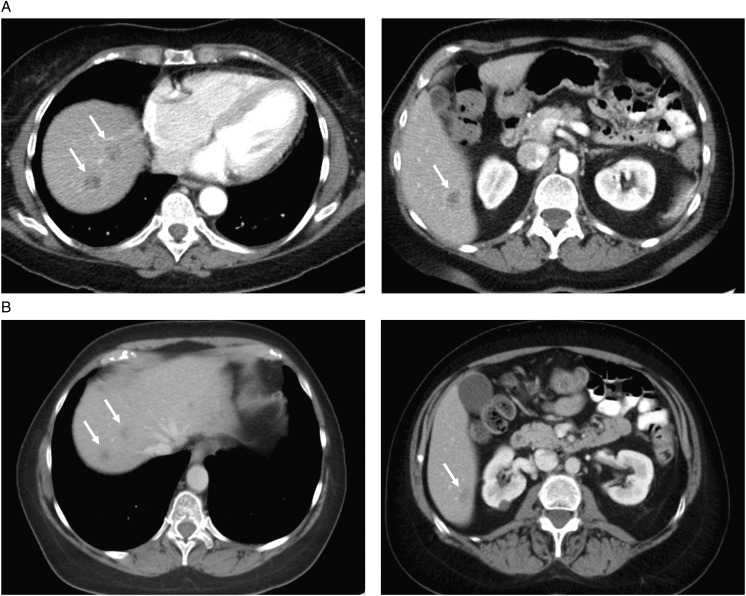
Computed tomography imaging. Computed tomography taken at (A) baseline before initiation of larotrectinib and (B) showing the best overall response of partial response to treatment with larotrectinib.
